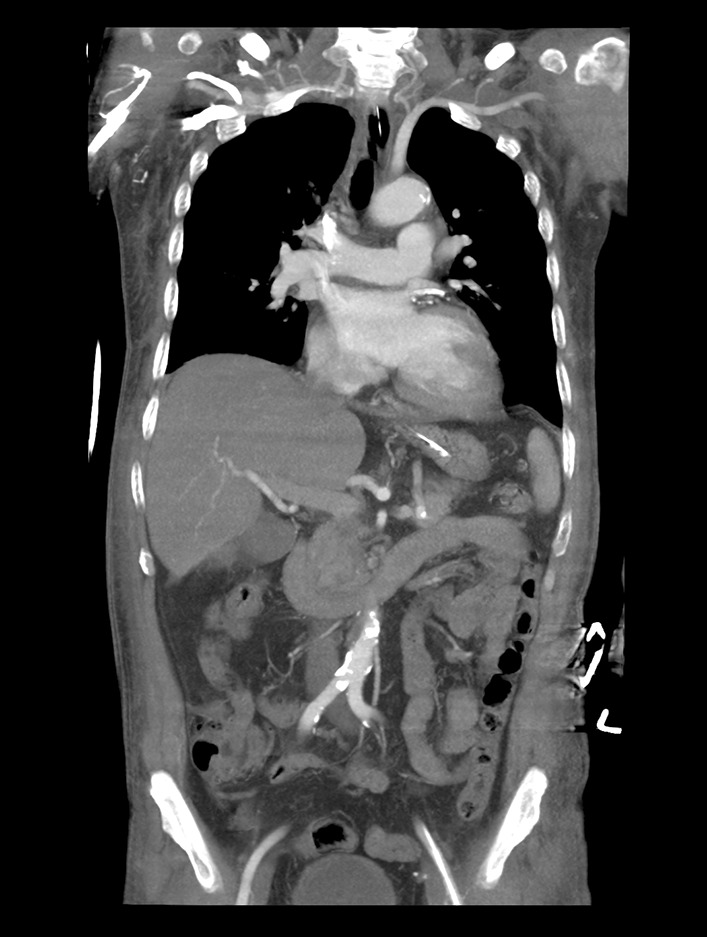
The final computed tomography angiography reveals no residual thrombus burden in both the pulmonary arteries
